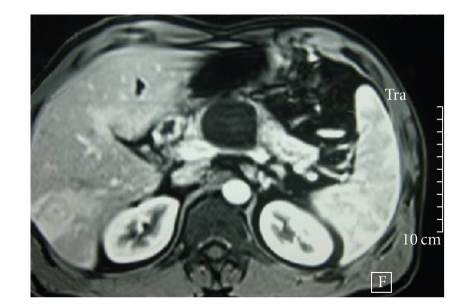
Pancreatic lesion and liver metastases on CT.
